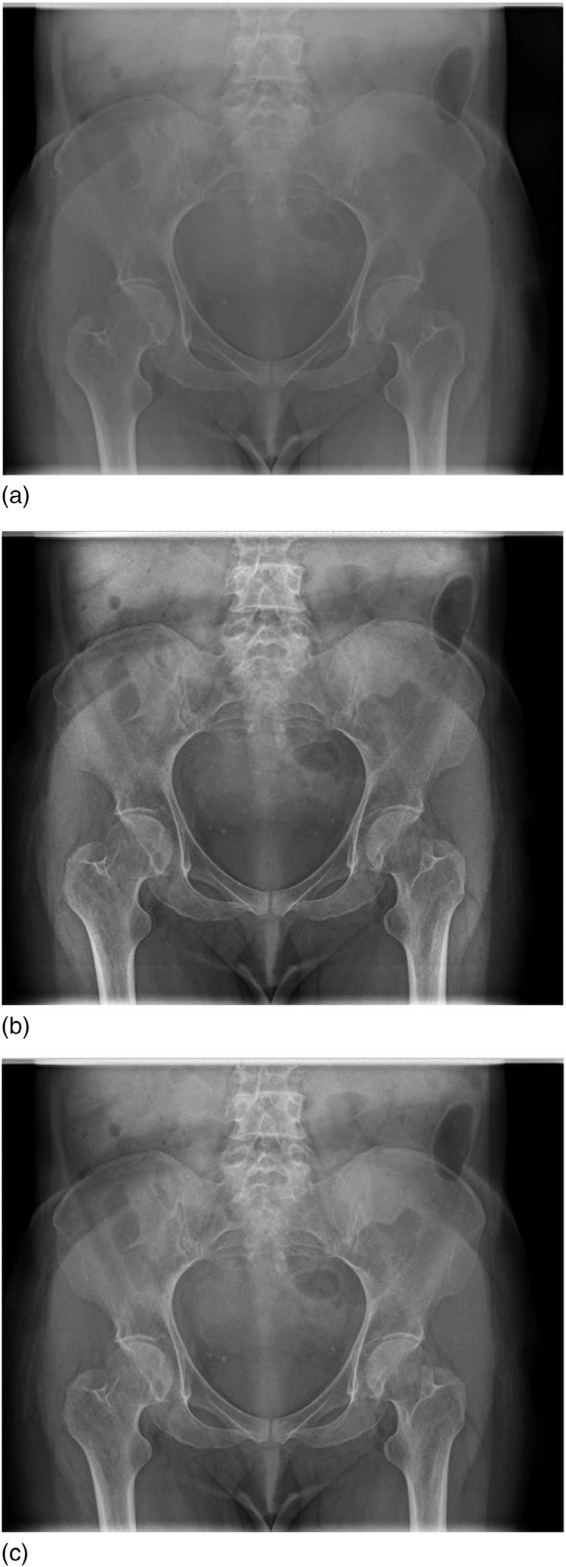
Example of the pelvic radiographs of the Thiel‐embalmed body. The presented radiographs were obtained with 81 kV, 2 mAs, and 0.1 mm Cu filtration. Figures (a–c) show the gridless, Virtual Grid SW ratio 10:1, and the physical grid radiographs, respectively.
